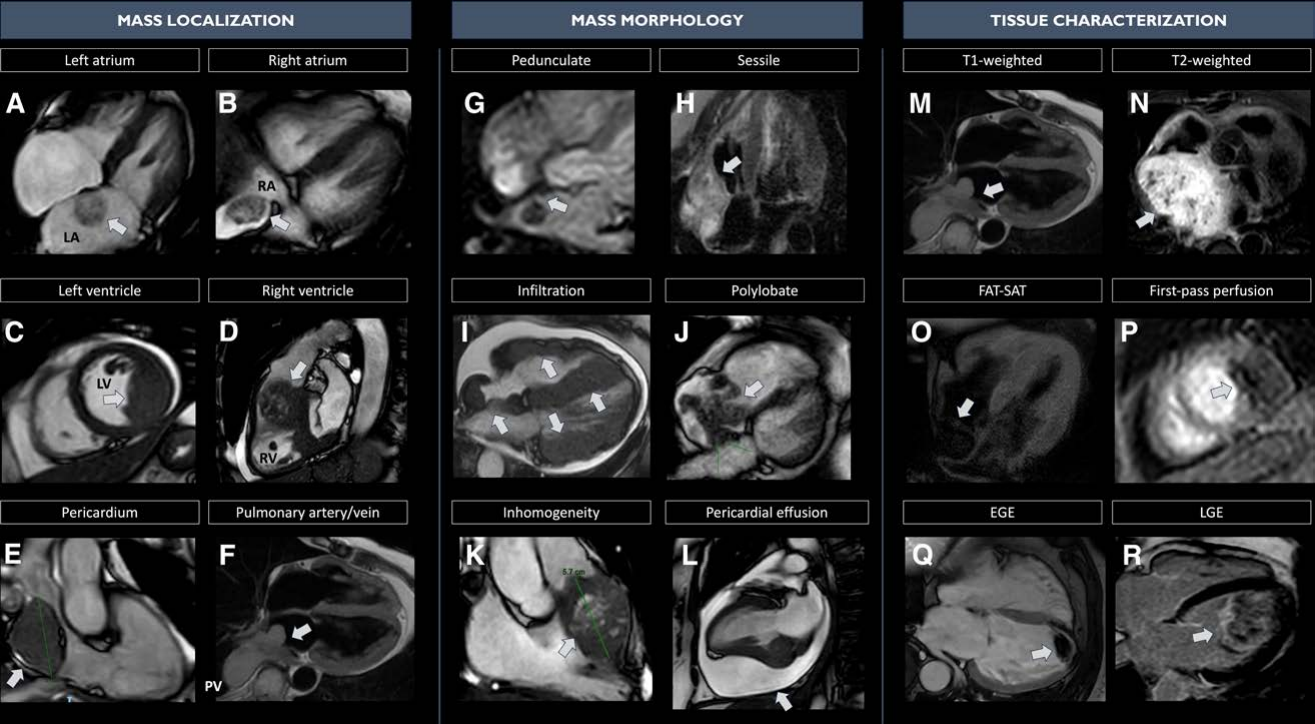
Case examples of cardiac masses features assessed at cardiac magnetic resonance (CMR) through a systematic approach. Cardiac masses (CMs) assessment at CMR should aim (at least) to evaluate mass localization, mass morphology, and tissue characterization. (I) Mass localization (A through F): examples of the most common localization of CMs (left atrium [myxoma], right atrium [neoplastic thrombus in patient with renal carcinoma], left ventricle [metastasis of melanoma], right ventricle [metastasis of urothelial carcinoma], pericardium [pericardial angioma], pulmonary artery/vein [atrial leiomyosarcoma]). (II) Mass morphology (G through L): examples of the CMs’ morphological features assessed (pedunculate [atrial myxoma], sessile [cardiac angiosarcoma], infiltration [aggressive B Lymphoma], polylobate [metastasis of hepatocellular carcinoma], inhomogeneous appearance [metastasis of urothelial carcinoma], pericardial effusion [metastasis of melanoma]). (III) Mass tissue characterization (M through R): Hypo/iso/hyperintensity at the following sequences: T1-weighted (atrial leiomyosarcoma), T2-weighted (pericardial paraganglioma), T1, fat saturation (FAT-SAT; lipoma of the right atrium), first-pass perfusion (metastasis of melanoma), early gadolinium enhancement (EGE; left ventricular thrombus), late gadolinium enhancement (LGE; metastasis of melanoma). T1w-TSE indicates T1-weighted turbo spin echo; and T2w-TSE, T2-weighted turbo spin echo.
